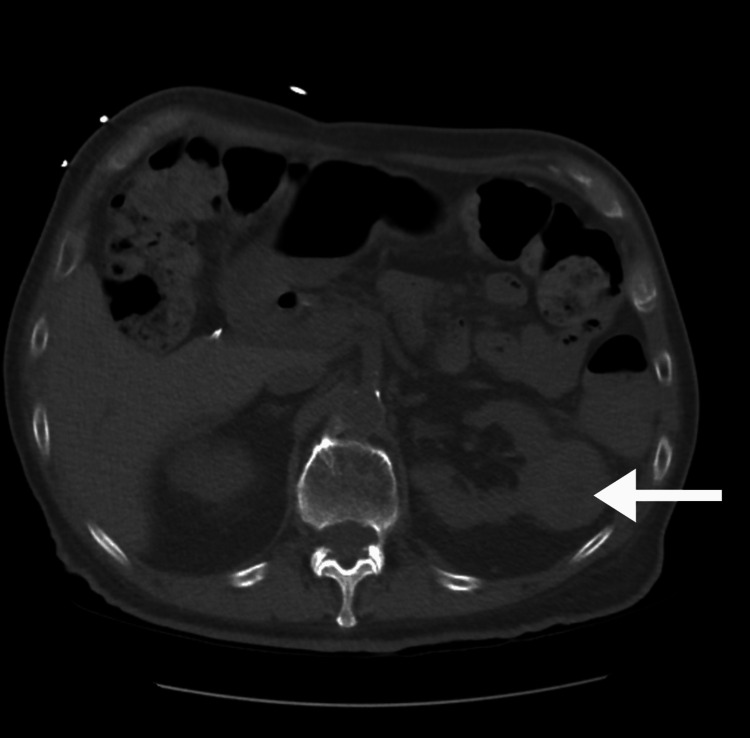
Computed tomography of the abdomen and pelvis showing a solid left renal mass (white arrow).
